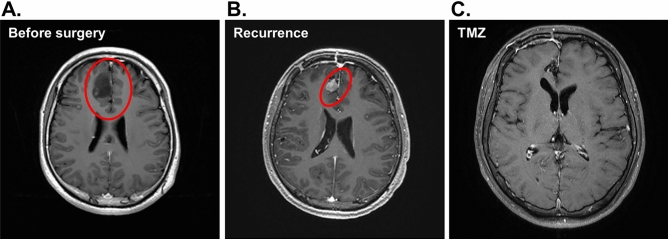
Radiological response of the brain lesion of patient #3 to temozolomide. (A) Axial MRI before surgery with the tumor area circled. (B) The follow-up MRI imaging after surgery. (C) Follow-up axial imaging after treatment by Temozolomide (TMZ). The patient showed complete response.
